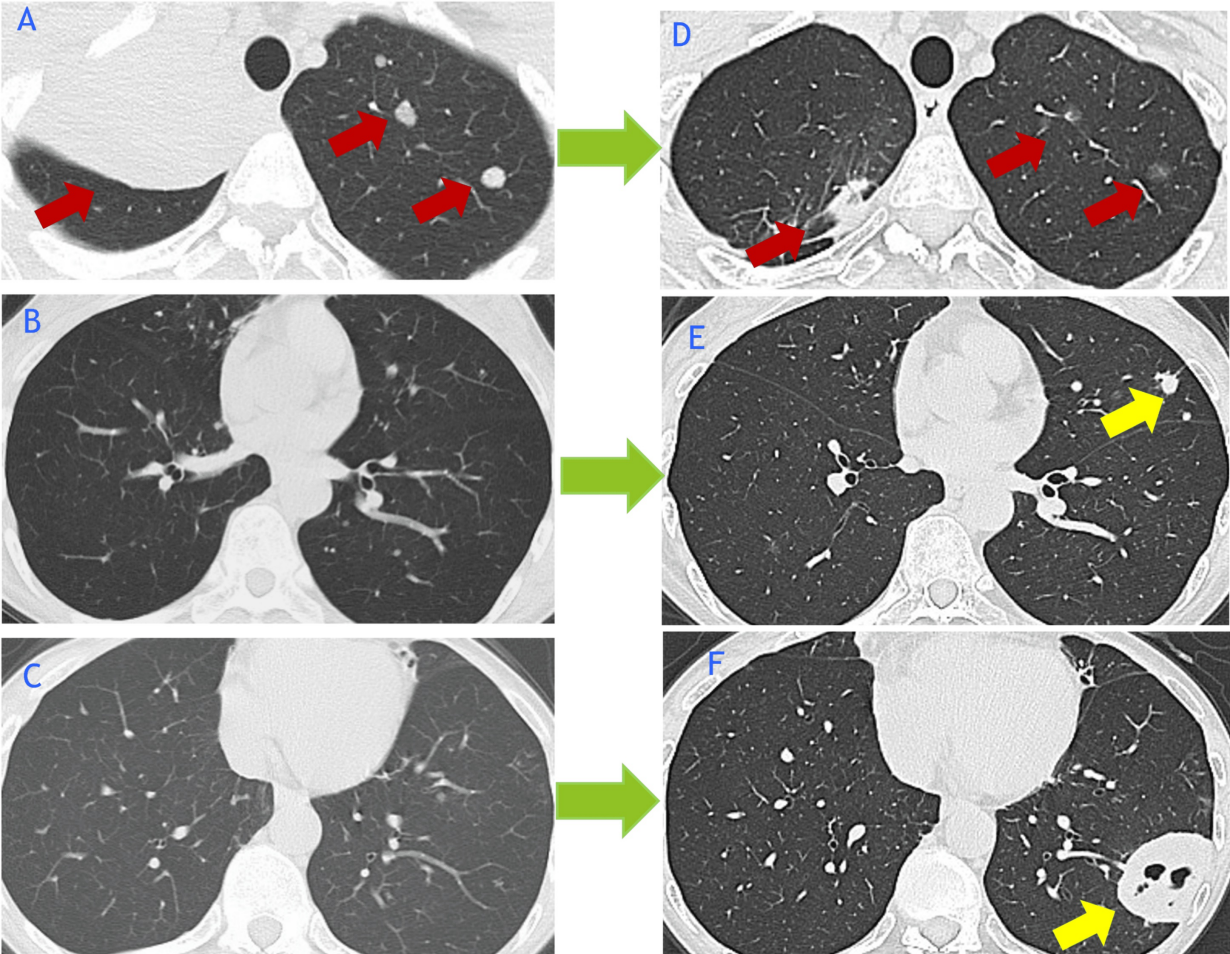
Chest CT findings before and after osimertinib treatment Chest CT images were obtained in X year Y-3 month (A–C) and Y month (D–F). Red arrows indicate tumor lesions that decreased in size following treatment with osimertinib. Yellow arrows indicate newly developed infiltrative shadows and cavitary lesions, suggestive of either disease progression or secondary infection. CT: computed tomography
